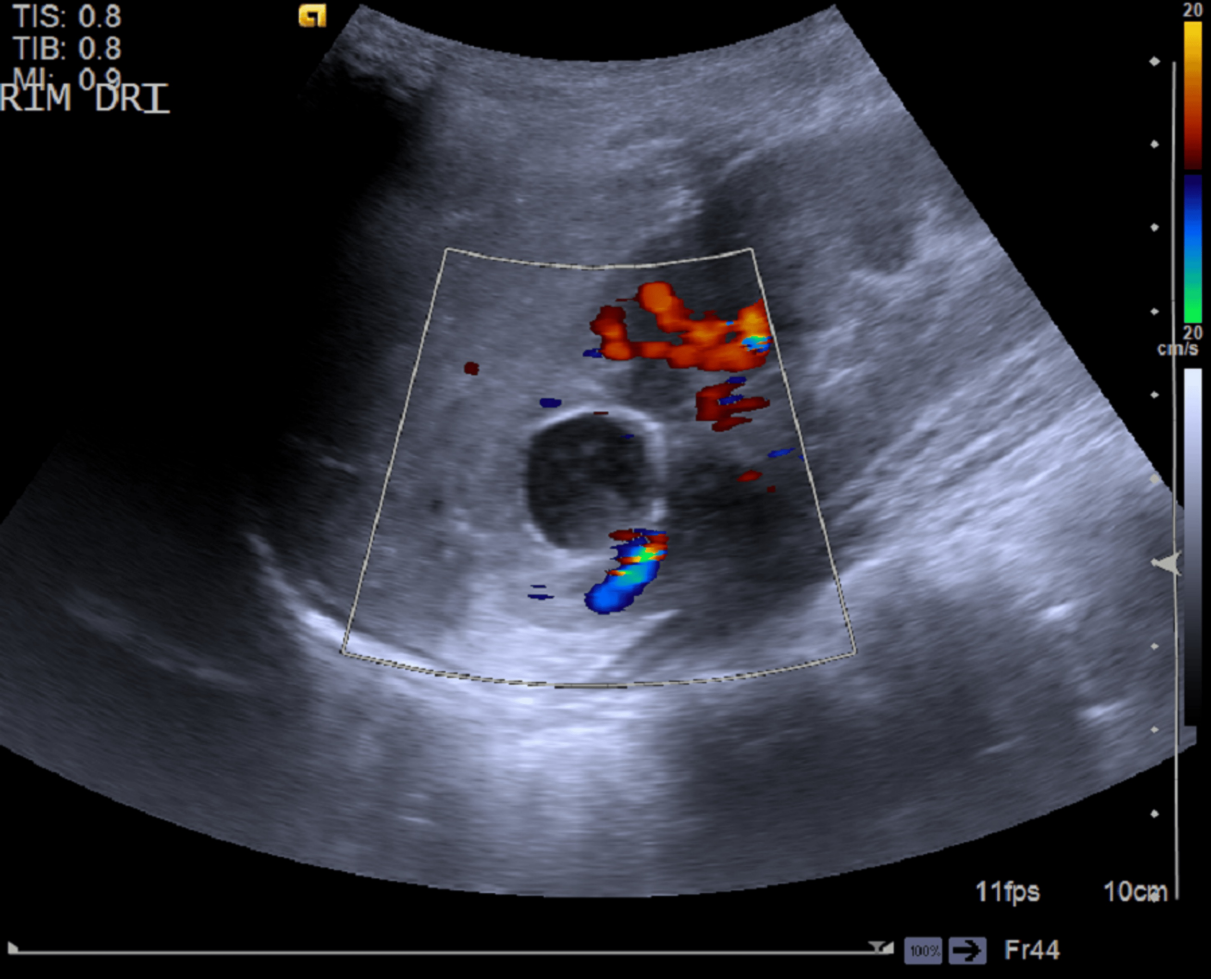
Ultrasound imaging showing a non-vascularized, thin-walled cystic formation with heterogeneous content in the upper third of the right kidney.
